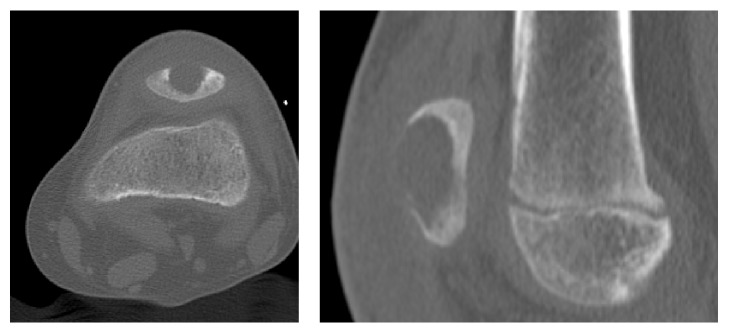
CT of the left knee clearly showing an osteolytic process with sharp borders.
